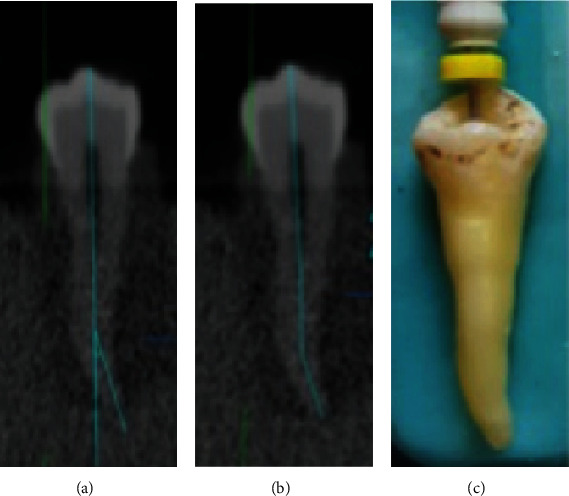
Representative digital image. (a) Canal curvature measurement. (b) Radiographic working length measurements obtained using CBCT imaging. (c) Clinical real length measurement of the same tooth.
